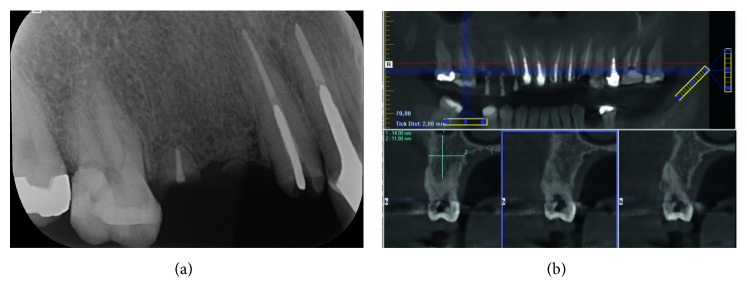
(a) Preoperative radiograph. (b) Upper maxillary CBCT of Tooth 1.6.
